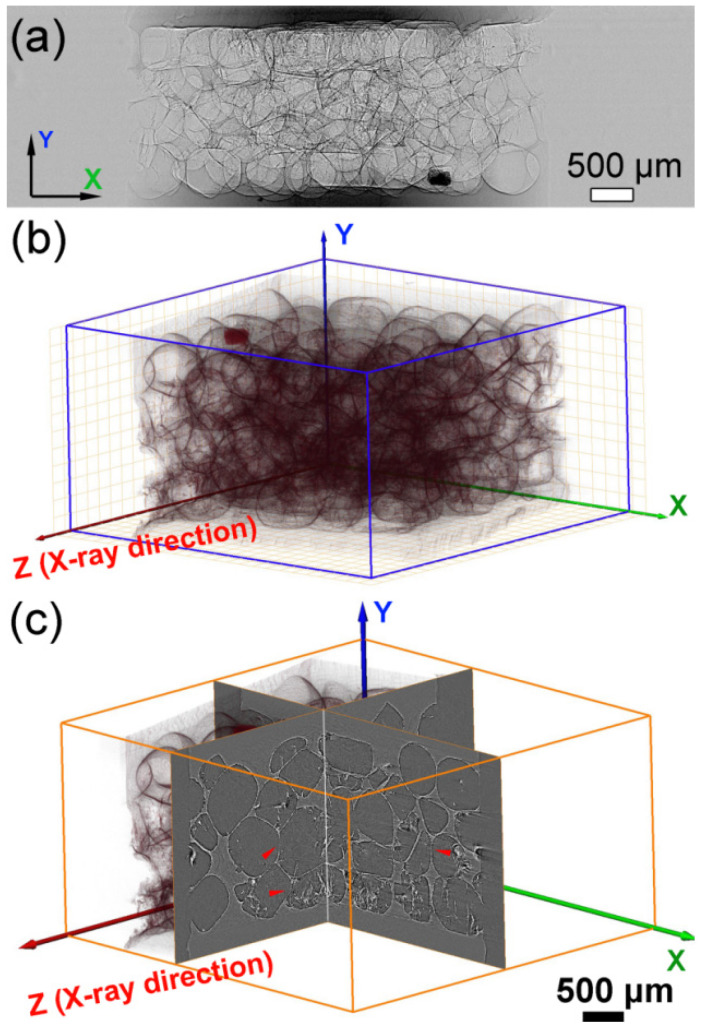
Synchrotron X-ray CT imaging of PEEK implants. (a) 2D projection image. (b) 3D morphological reconstruction. (c) Tomographic slice analysis showing sandwich-like structure and pore connectivity.
